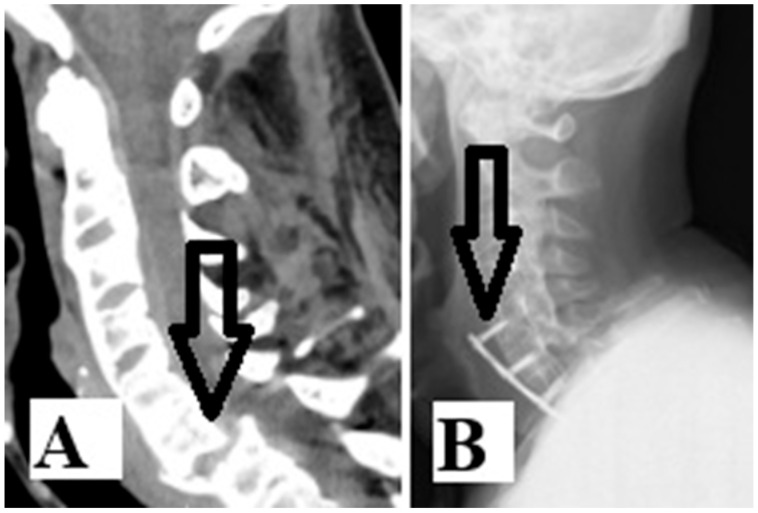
Sagittal CT scan (A) revealed a luxation fracture at the C6–C7 level (arrow). Follow-up X-rays scan after six months (B) revealed hardware failure (arrow), which led to the esophagus injury.
